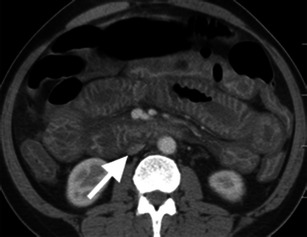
Shock bowel. Contrast-enhanced axial CT image demonstrates diffuse bowel wall thickening with mural hyper-enhancement. Note the secondary sign of a flattened inferior vena cava, indicating hypovolaemia
